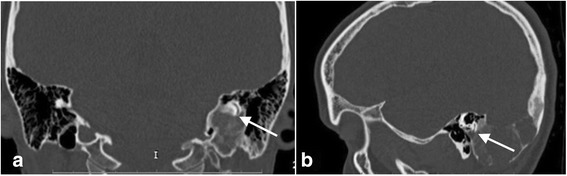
a Coronal CT scan shows a fistula of the posterior semicircular canal. b Sagittal CT scan shows extensive involvement of the petrous apex of the temporal bone by fibrous dysplasia and involvement of the labyrinth
